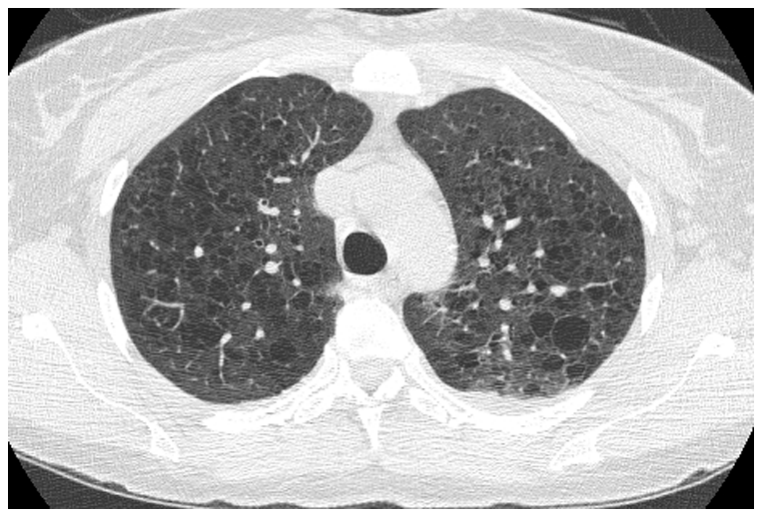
Selected CT image showing emphysema in HIV patient.
